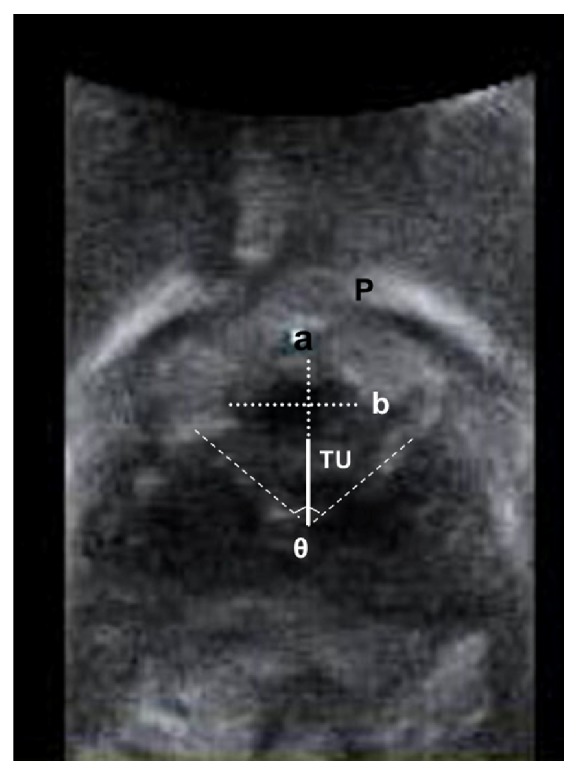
Ultrasound image (axial plane) of the midurethral hypoechoic core in a woman with tension-free vaginal transobturator tape, showing the measurements made for this study: the angle between the two arms of the sling (*θ*), the shortest (a) and longest (b) diameters of the urethral hypoechoic core and the distance between tape and urethra (TU). P, pubis symphysis [12].
